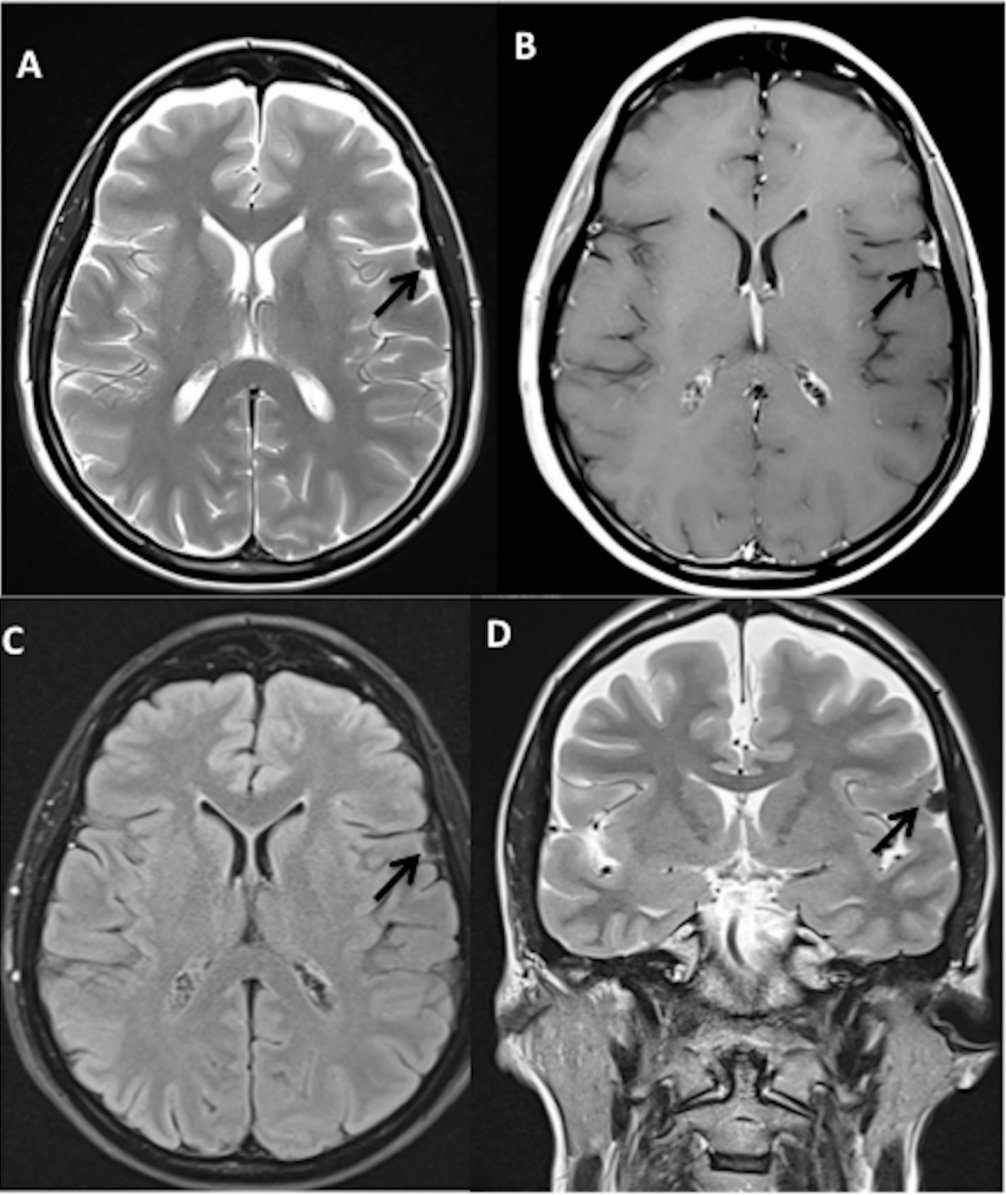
Preoperative magnetic resonance imaging of the brain showing left frontal meningioma (patient 1) Black arrows point to the lesion. A) Axial T2, turbo spin echo, B) Axial T1 post contrast, C) Axial T2, fluid-attenuated inversion recovery, D) Coronal T2
